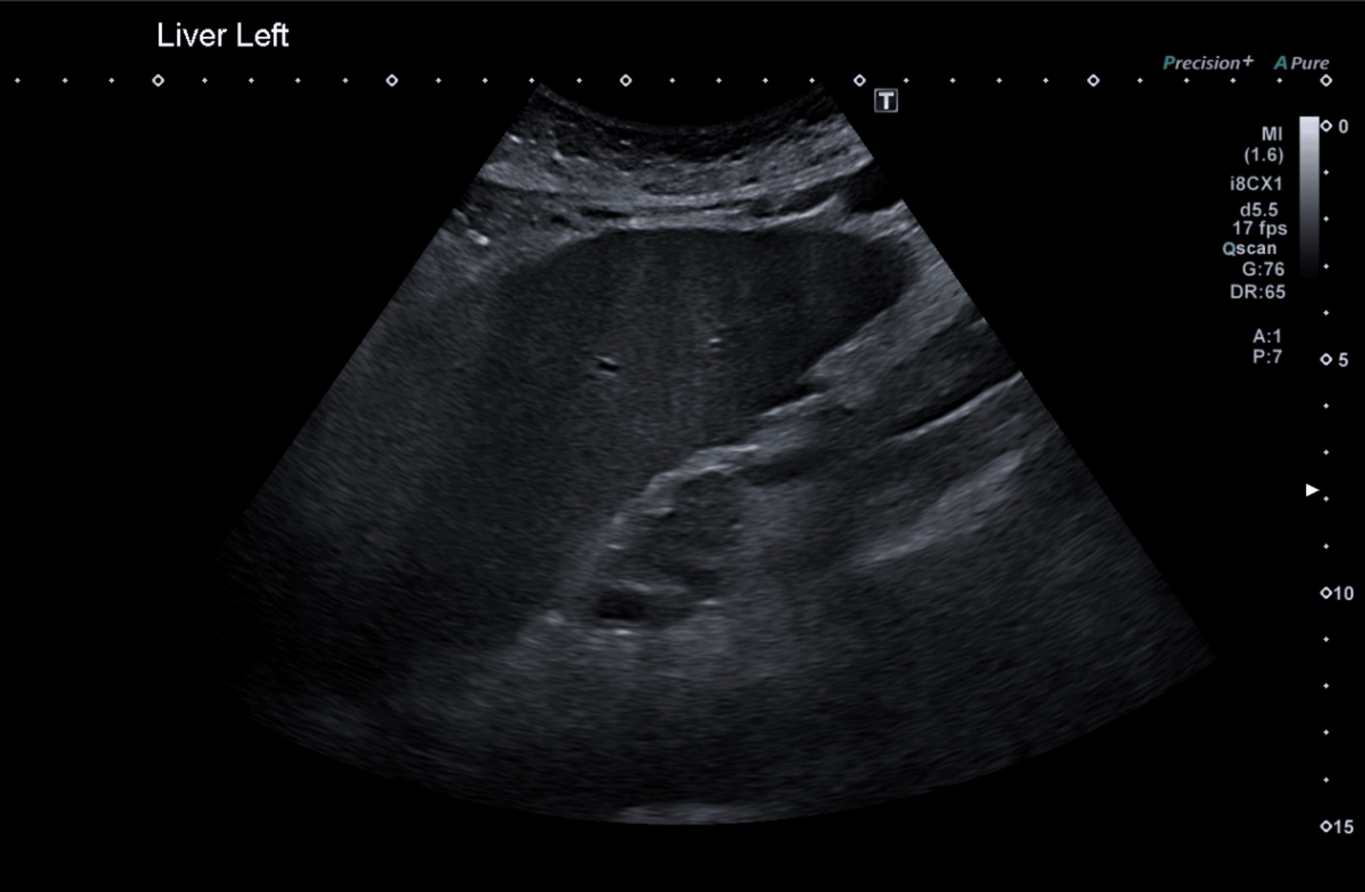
Ultrasound showing moderately enlarged cirrhotic liver and a thick-walled gallbladder without calculi or sludge.
